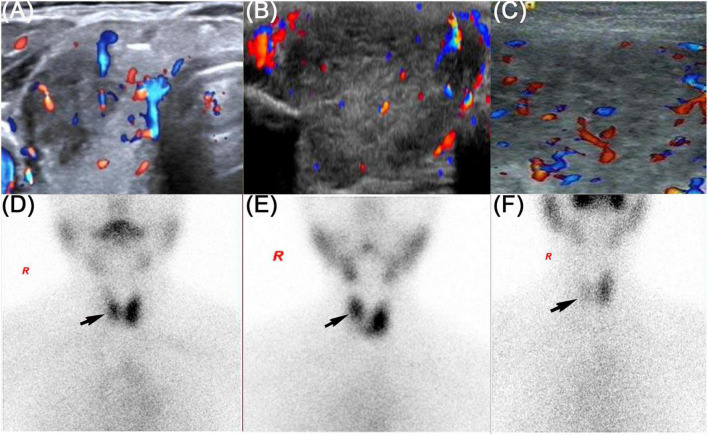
Thyroid ultrasound and thyroid emission computed tomography of three patients. The black arrow showed the radioactive distribution defects in the thyroid lobe. (A,D) Refers to patient 1, (B,E) refers to patient 2, and (C,F) refers to patient 3.
